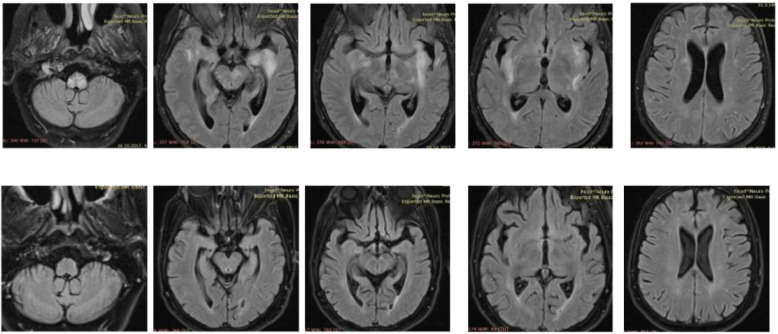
Brain MRI: multiple parenchymal supratentorial and brainstem demyelinating lesions on T2 and FLAIR sequences without postcontrast enhancement on T1WI (upper row); follow-up MRI six months after hospitalization showing complete regression (bottom row).
